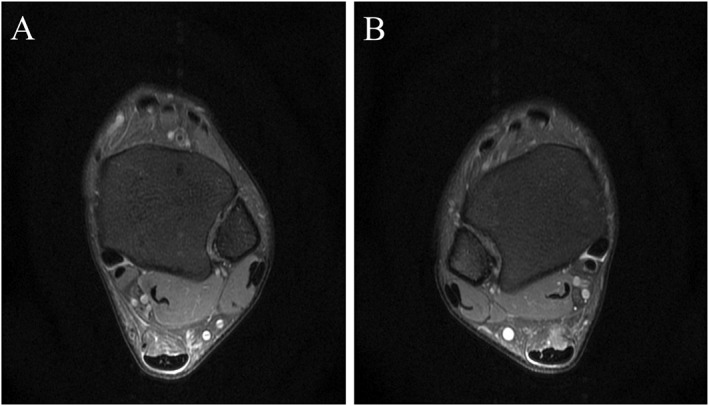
A 37‐year‐old male amateur marathon runner with a 3‐year running experience and a monthly running distance of 200 km; representative MRI images of paratendinopathy. (A–B) Axial fs‐PDWI of bilateral ankles shows circumferential hyperintense signal enhancement around the Achilles tendon.
